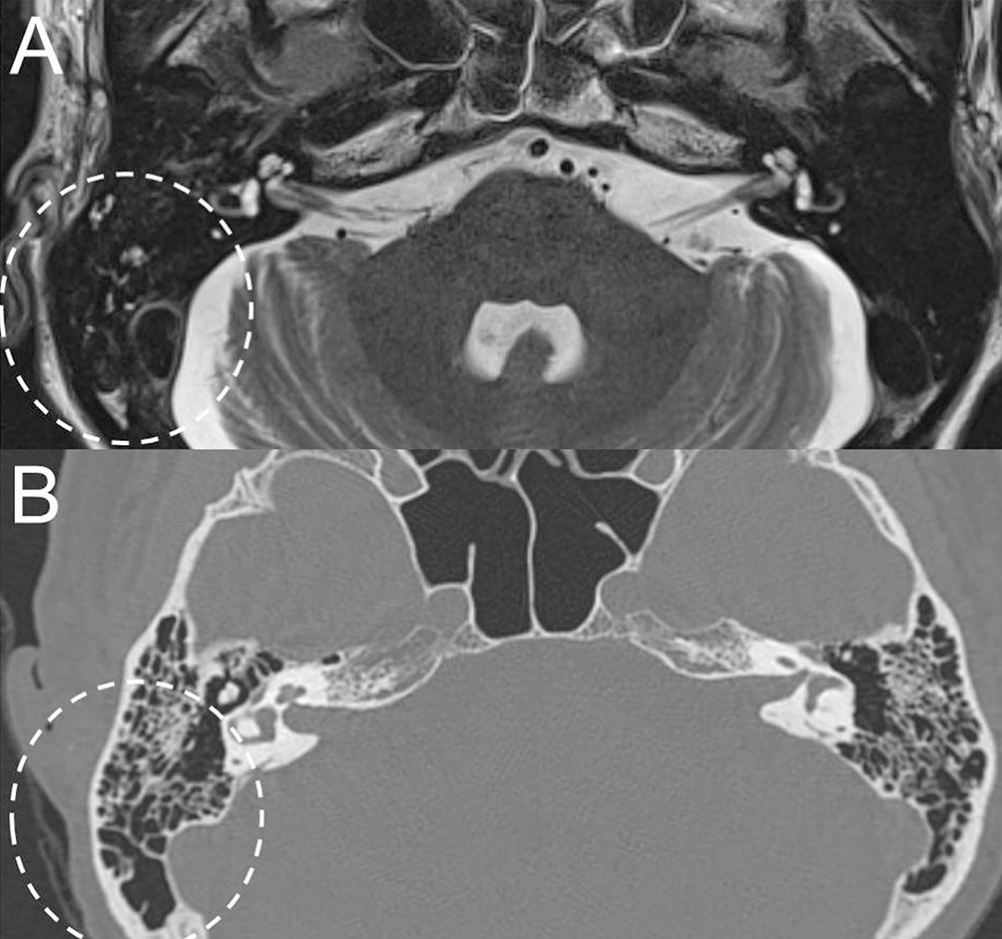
Comparison between temporal bone MRI and TBCT in a representative case of Ramsay Hunt syndrome with mastoid effusion on the right side. Non-contrast axial T2-weighted image at the level of IAC and mastoid air cells shows high signal intensity from the fluid in the right mastoid suggesting mastoid effusion (dotted circle, A), which was not easily identifiable in TBCT (dotted circle, B).
